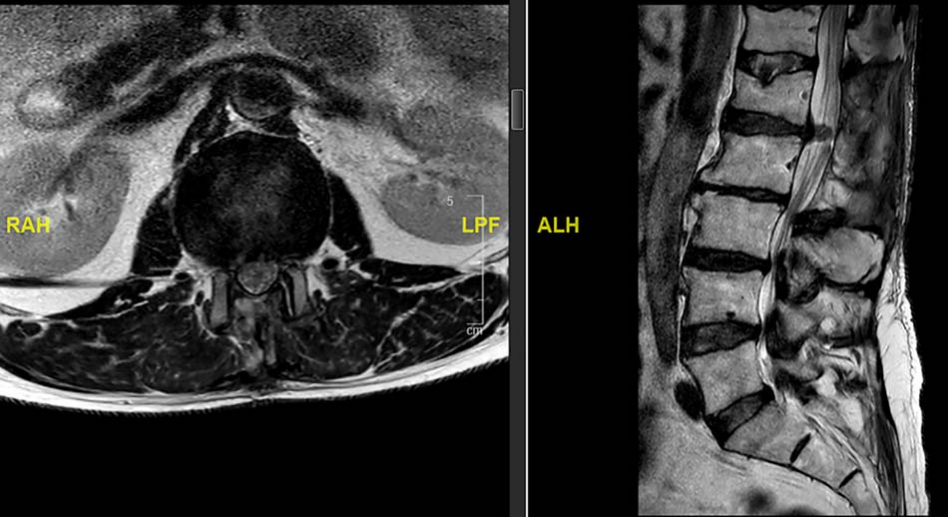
MRI image revealing a recurrence of a disc prolapse, suspected to be an intradural disc at L1/2, confirmed intraoperatively.
